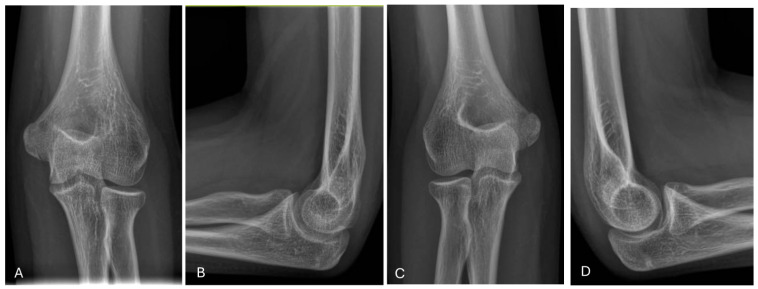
Radiographic follow-up after 73 months after surgery. (A) Anteroposterior view of the affected elbow. (B) Lateral view of the affected elbow. (C) Anteroposterior view of the contralateral elbow (D) Lateral view of the contralateral elbow.
